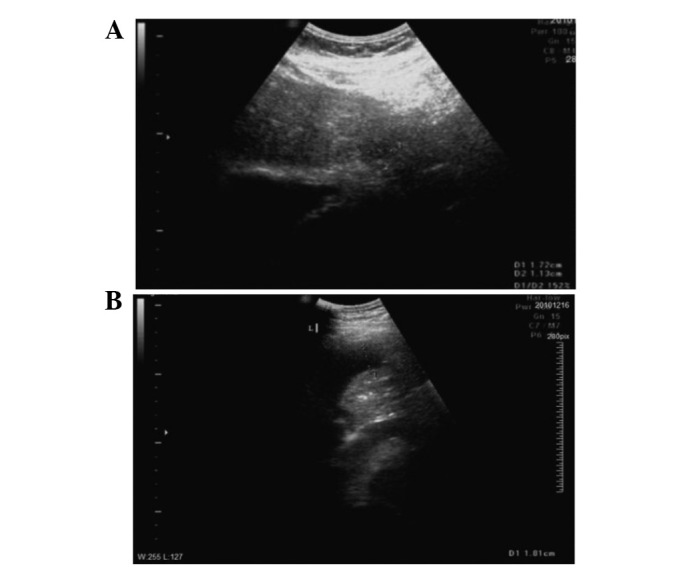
(A) Intraoperative B-mode ultrasound showing a weakened echo in a 17×11-mm area in the left lobe of the liver. (B) Post-operative B-mode ultrasound showing a filling defect in the radiofrequency ablation (RFA) area.
